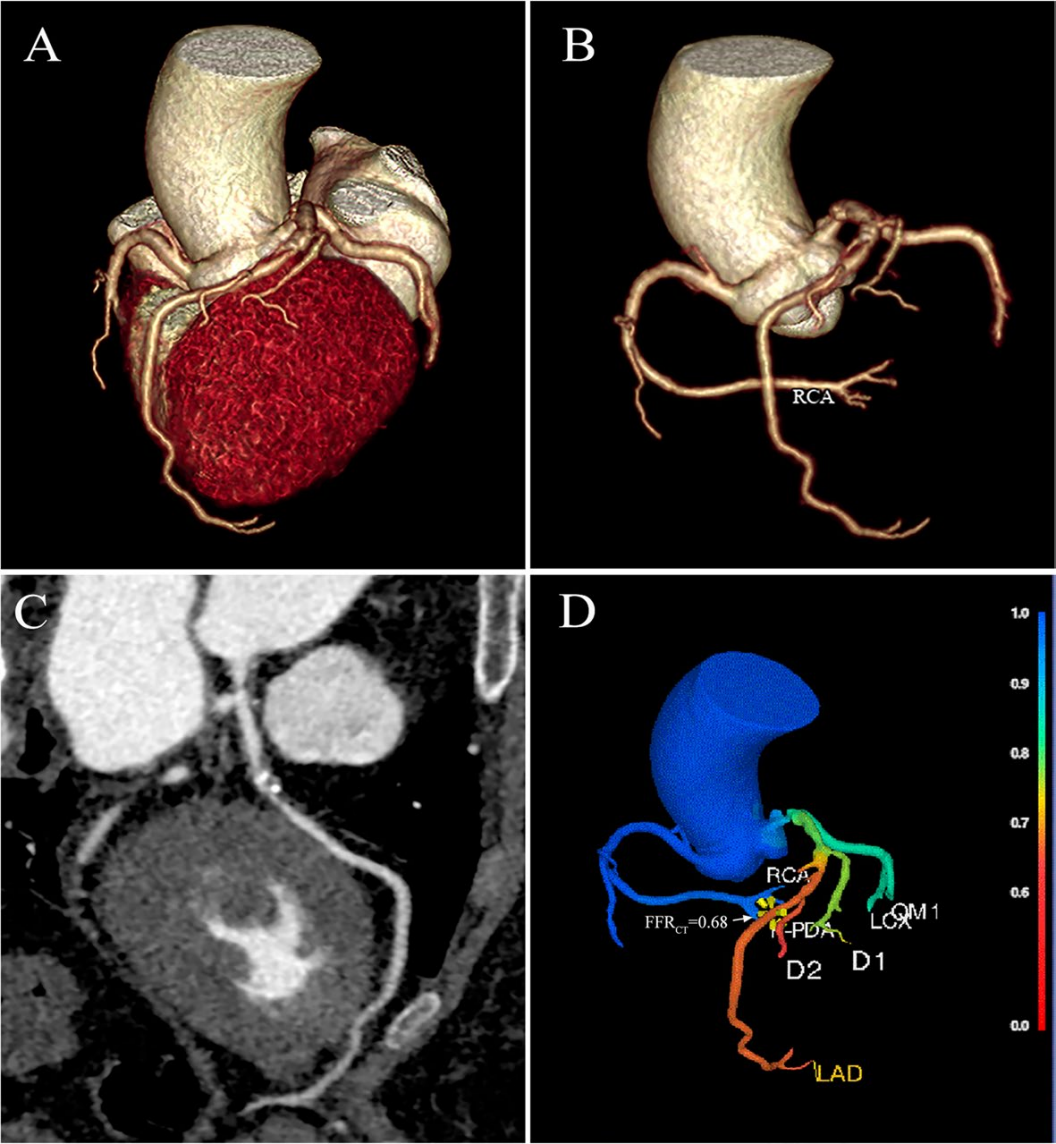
Example case. The left anterior descending artery contained mixed plaques with functional ischemia: 3-dimensional (volume rendering) (A, B), curved planar reconstruction (C) and coronary computed tomography angiography derived fractional flow reserve [the measurement location (yellow marker and white arrow): 2 cm distal to the stenosis in plaque artery]. (D)
